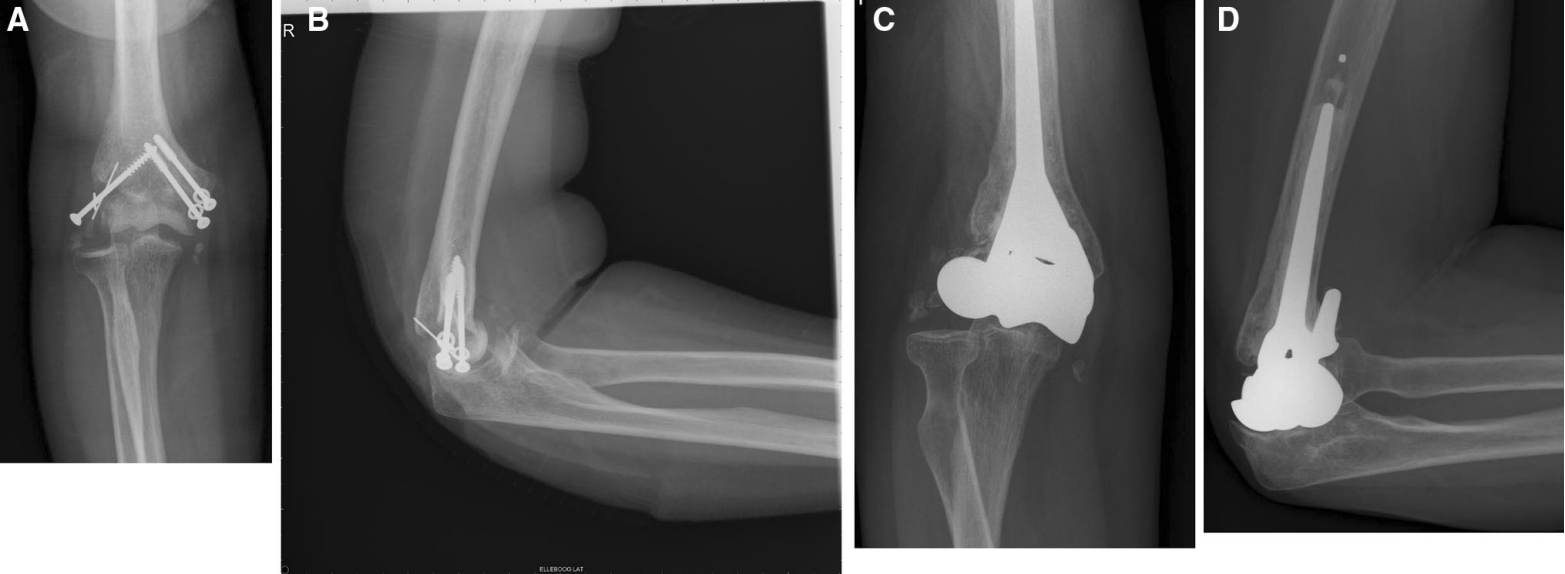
a–d Antero-posterior and lateral radiographic image of the elbow before (a, b) and at end follow-up (c, d) 61 months after distal humerus prosthetic hemiarthroplasty (humeral component of Latitude^®^ Total Elbow with anatomical spool) of case 2. Subluxations of the ulnohumeral joint and discrete irregularity of the articulating surface of the proximal ulna are noted
